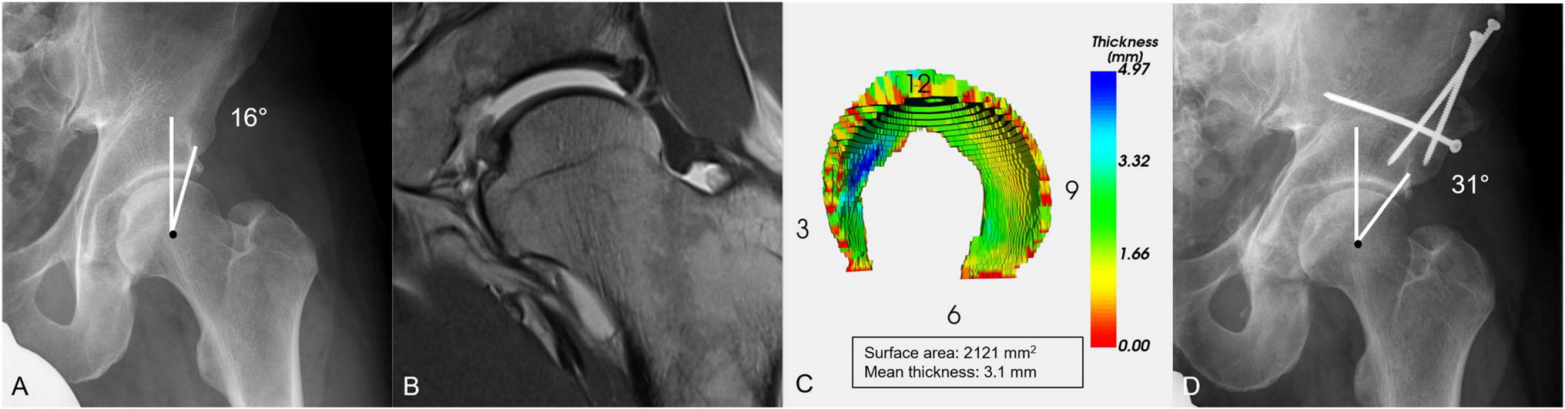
A Patient with hip dysplasia (lateral centre edge angle of 16$$^\circ $$). B Hip MRI indicated reduced acetabular coverage. C 3D cartilage model with colour-coded means of cartilage thickness shows a small surface area of 2121 mm$$^2$$ and a high cartilage thickness, typically seen in hip dysplasia. D Periacetabular osteotomy was performed to increase acetabular coverage (lateral centre edge angle = 31$$^\circ $$), i.e. to correct for hip dysplasia
